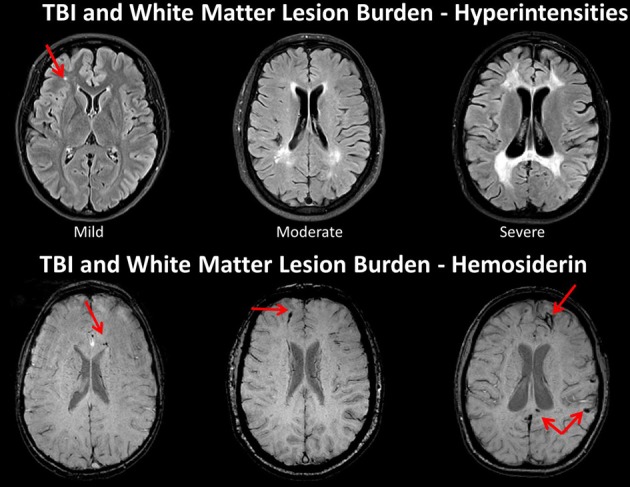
TOP: Fluid attenuated inversion recovery (FLAIR) sequence in three traumatic brain injury (TBI) cases depicting different levels of white matter burden. (Left) a child with mild TBI (mTBI) indicating a solitary, focal white matter hyperintensity (WMH). (Middle) a 62-year-old male with a severe TBI with no white matter abnormalities noted on admission CT. Patient had a GCS of 7 prior to intubation, meeting criteria for severe TBI (Right) 17-year-old injured 2 years prior with an admission GCS of 3. Note the prominent and extensive WMHs widely distributed. BOTTOM: The middle and right hand subjects are the same as above, but subject on the left side is a different child with a mild TBI, who did not have a WMH, but did show hemosiderin in the corpus callosum (arrow). Note that both patients with severe injury have some generalized atrophy and ventricular dilation as a reflection of generalized brain volume loss as a consequence of severe TBI along with multiple hemosiderin deposits.
